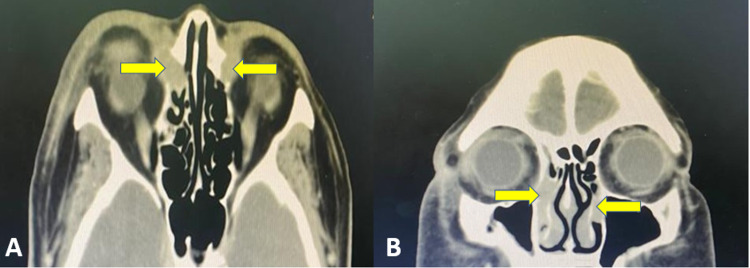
A) Axial and B) coronal view of CT scan showing a homogenous soft tissue mass in the lacrimal sac which is isodense with respect to extraocular muscle (yellow arrow).
